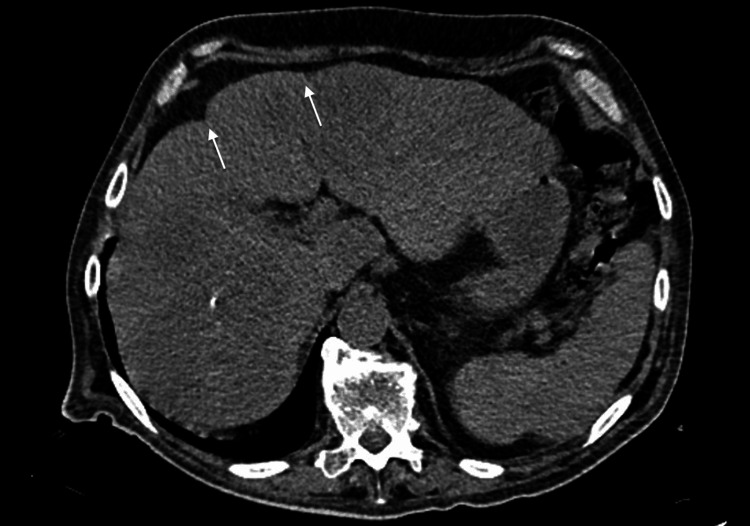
CT scan showing the liver with irregular contour (white arrows) CT: computed tomography
